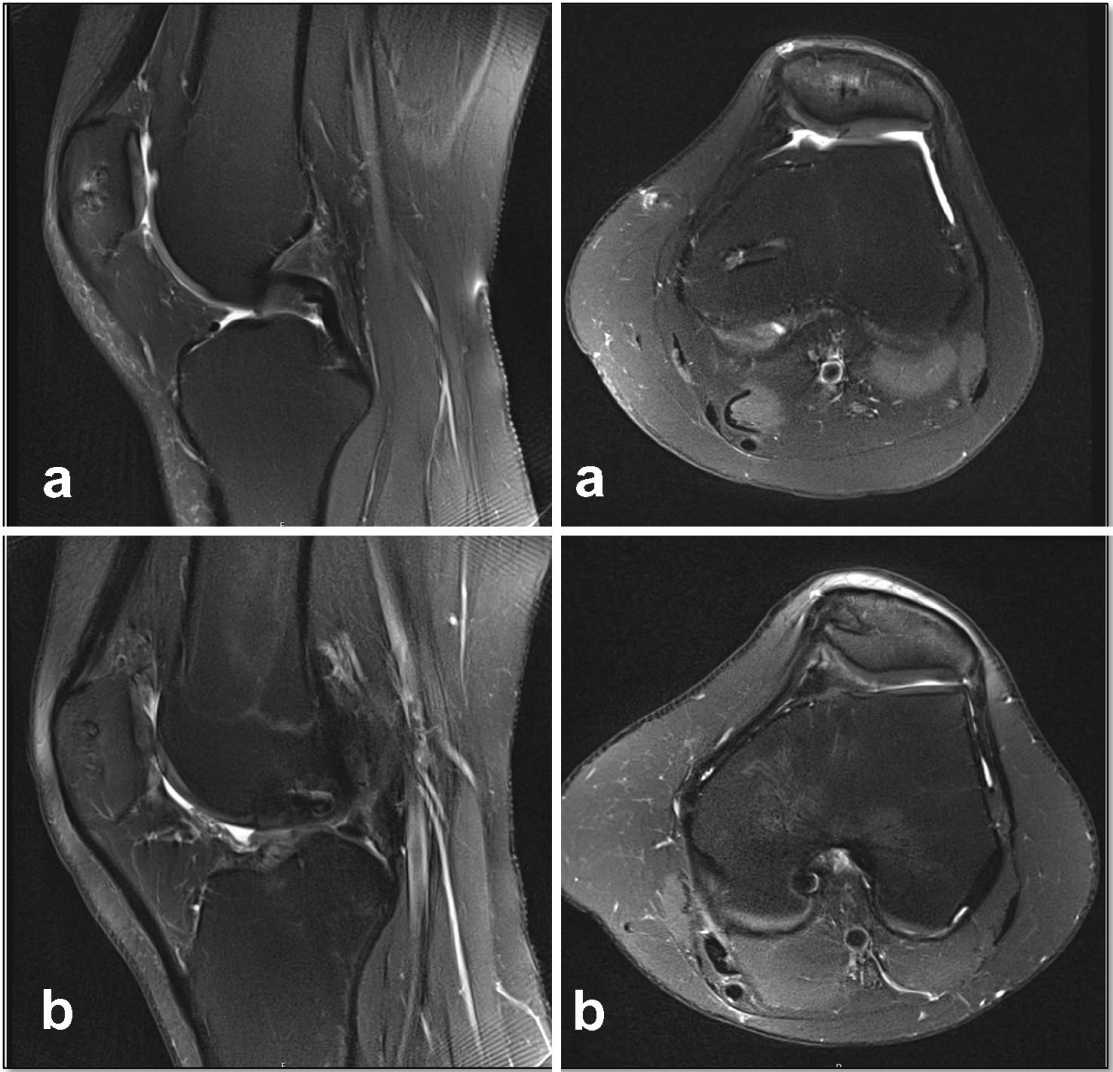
MR images at follow-up after open refixation (a) and ACI (b) show results after surgery with good integration of chondral tissue. Images after refixation (a) show good integration of the flake fragment after completed healing reaction. Images after ACI (b) show a good integration of the transplanted chondral tissue, with minor hypertrophy
